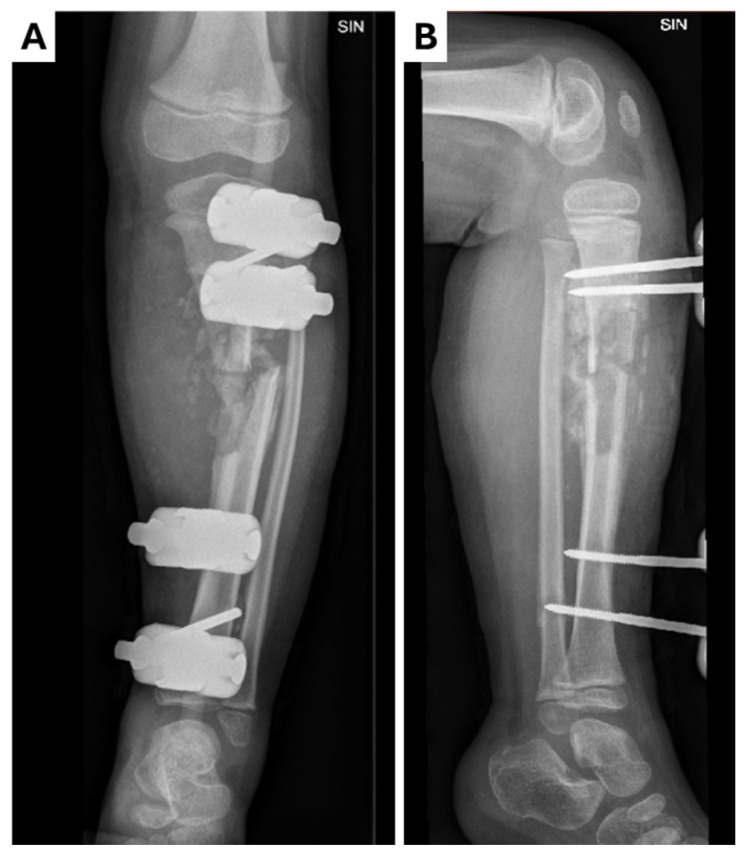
Control X-ray of left leg in (A) anterior–posterior and (B) lateral projections after surgical manipulation and external fixation. During surgery, the rotation of the medial part of the tibia toward the fracture was performed along with the drainage of pus (~500 mL) around the fracture site. Pseudoarthrosis was observed during surgery along with the presence of infected cartilage tissue. Resection of the damaged bone was performed, resulting in limb shortening (~1 cm). A chlorhexidine impregnated material was left in the wound.
